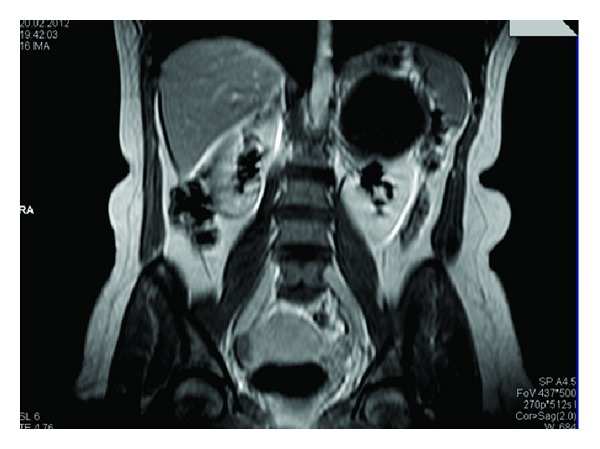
T1-weighted MRI image of adrenal lymphangioma in coronal section.
